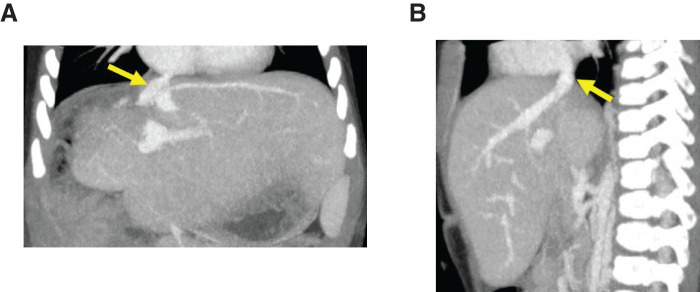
(A, B) Contrast-enhanced CT scan with coronal and sagittal plane showed the absence of tumor recurrence and patency of the left hepatic vein reconstructed by the ePTFE graft (arrow). ePTFE, expanded polytetrafluoroethylene
